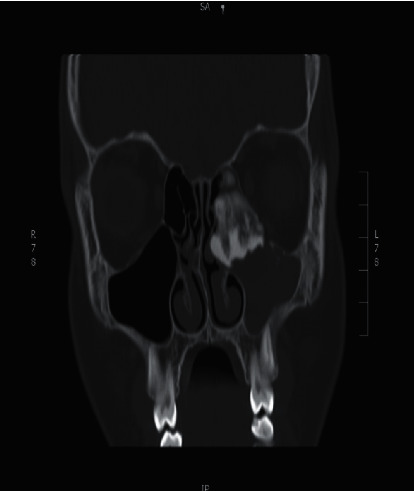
Computed tomography of paranasal sinuses in the coronal plane, showing a left ethmoid calcified soft tissue mass with inferior extension into the superior aspect of the left maxillary antrum.
